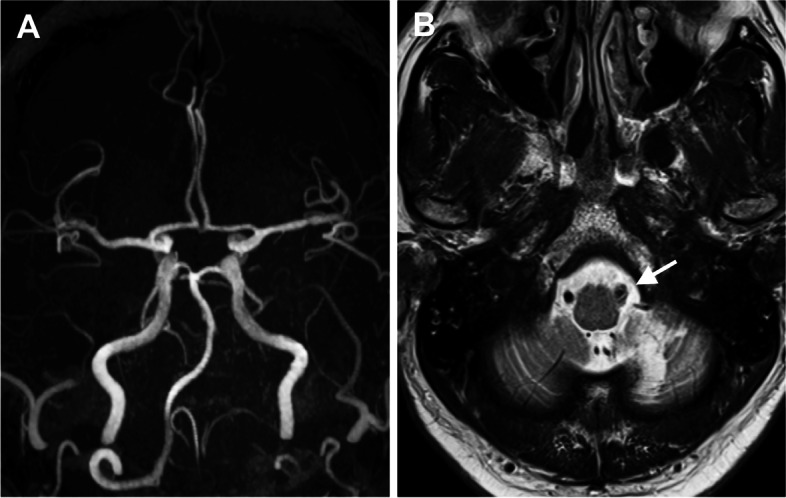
Magnetic resonance (MR) images on admission (Case 1). A Time-of-flight MR angiography revealing occlusion of the left vertebral artery. B T2-weighted image mimicking left vertebral dissection (arrow)
